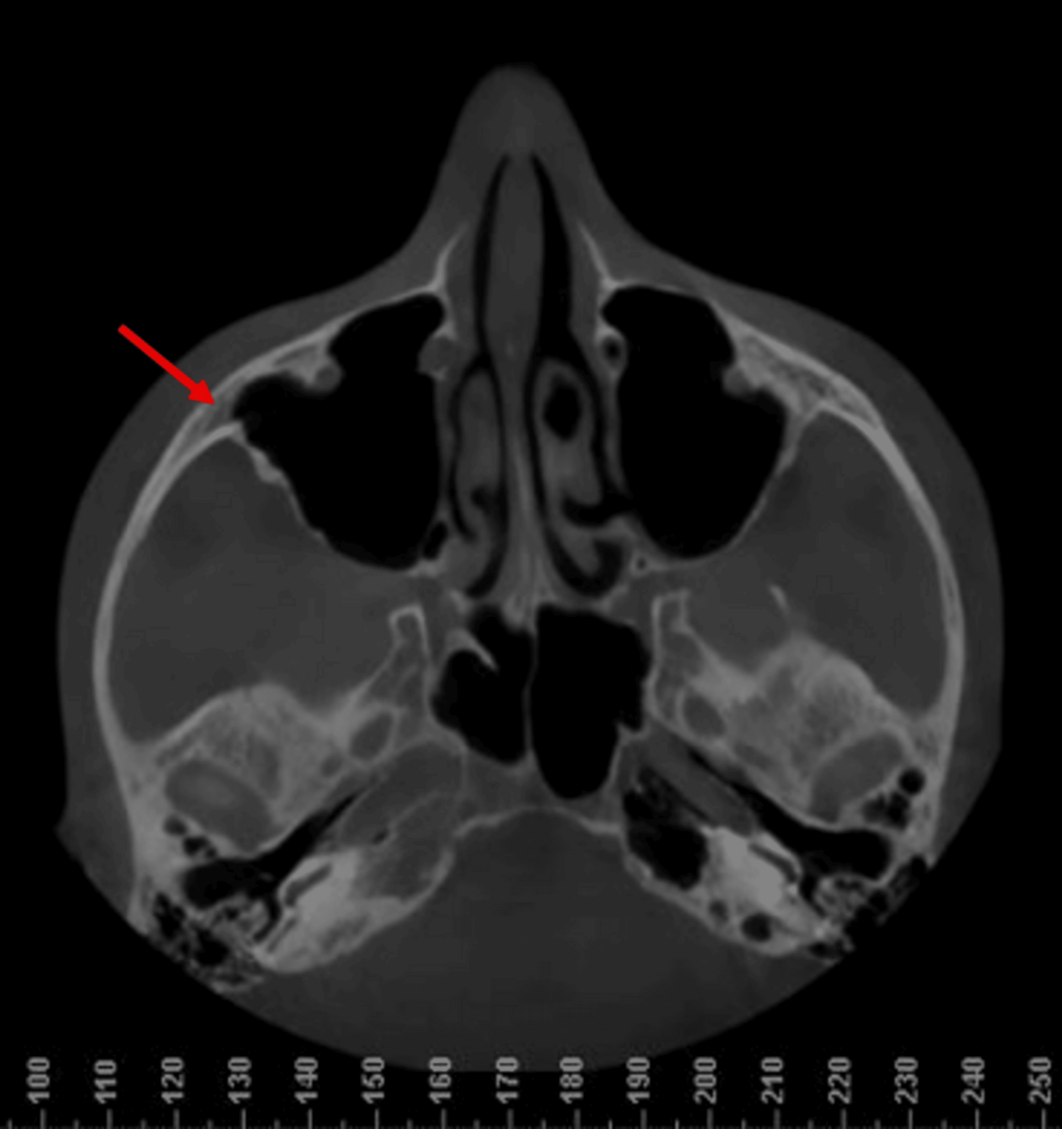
Axial slice on a CBCT indicating the exact location of the zygomaticomaxillary suture (red arrows). CBCT: Cone-beam computed tomography.
